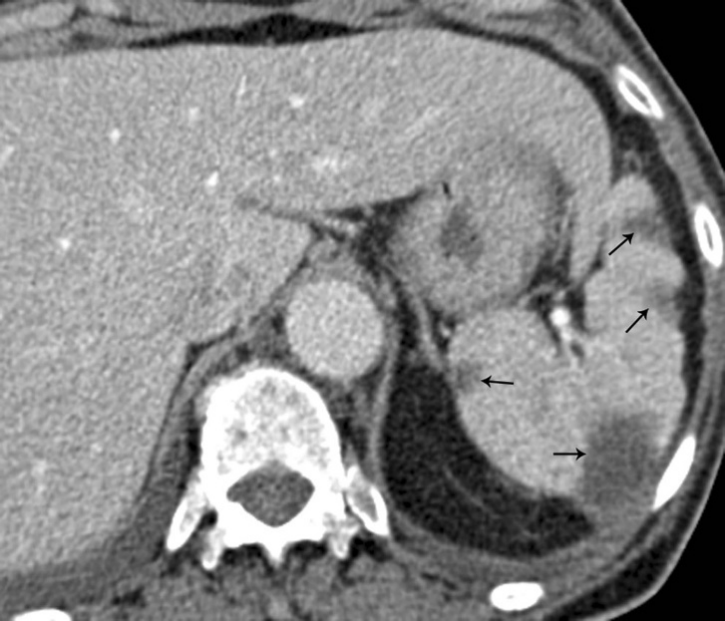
Abdominal contrast-enhanced CT shows multiple hypodense lesions in the spleen (*black arrows*). All these lesions are triangular with their broadest base on the periphery of the spleen
